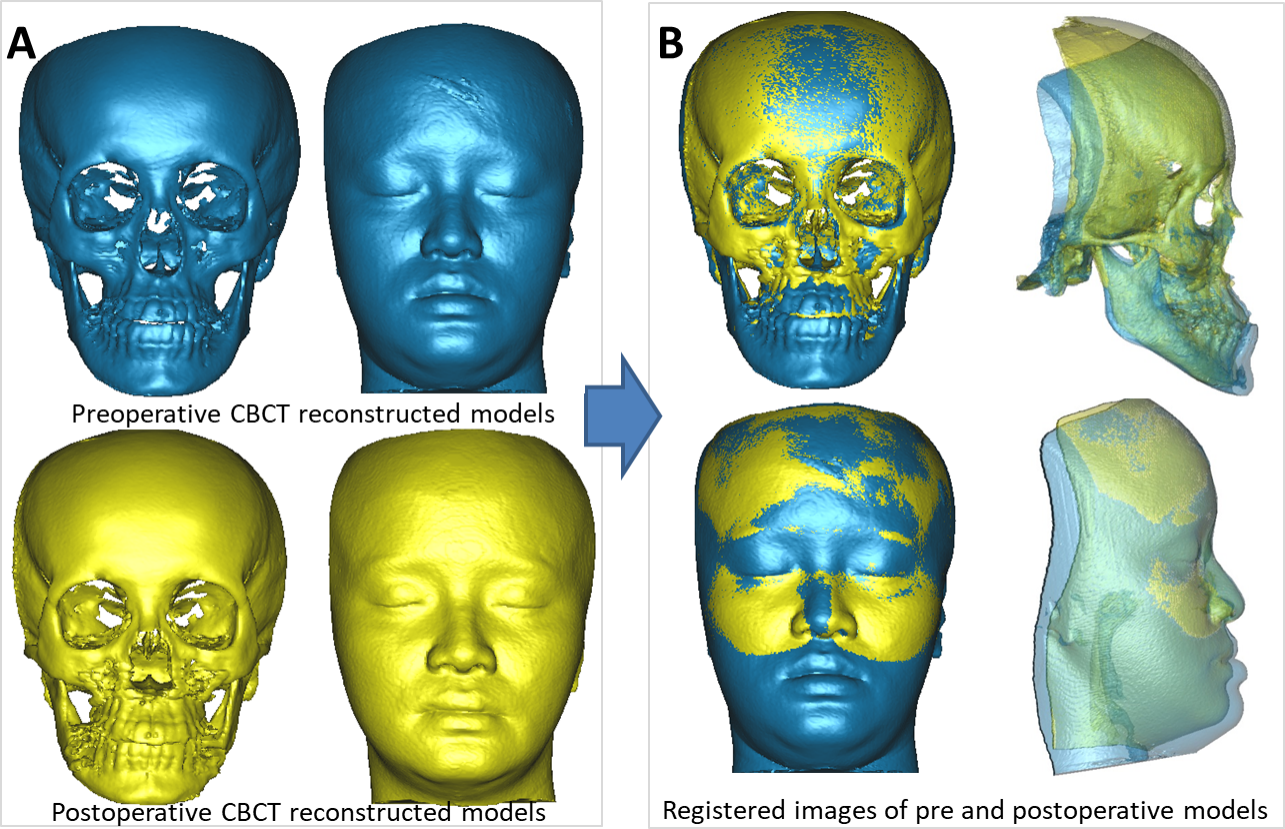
Cranial base registration of preoperative and postoperative CBCT images (case no. 21). 3D preoperative and postoperative models (A). Registration of preoperative and postoperative CBCT models (B).
